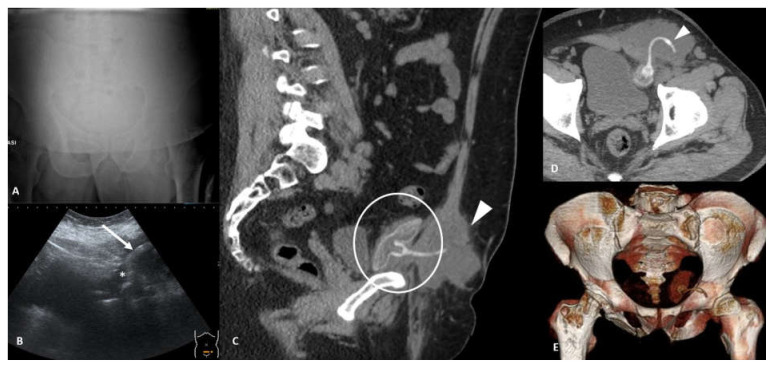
Fluid collection. Fifty-two-year-old male with history of IPP (inflatable penile prosthesis) implantation 6 years ago and recent onset of left groin pain. Frontal orthostatic radiogram (A) does not show pneumoperitoneum. Ultrasound pelvic scan (B) shows hyperechoic complex image (arrow) in left iliac fossa within a hypoechoic collection (*). Sagittal contrast-enhanced CT (C) shows IPP reservoir placed in the retropubic space of Retzius and rectus abdominis tumefaction (arrowhead). Axial non-contrast CT image (D) better shows left rectus abdominis tumefaction caused by reservoir saline loss due to disruption of IPP connecting tube (arrowhead); CT 3D-reconstruction (E) shows reservoir integrity and connecting tube disruption.
